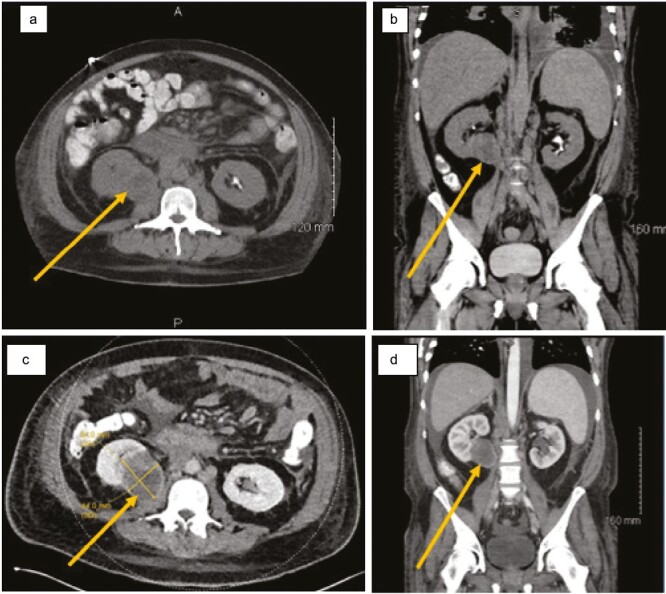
Computed tomography of right kidney mass at 2 timepoints. Axial (Panel A) and coronal (Panel B) CT at the time of initial workup demonstrated a mass in the right lower pole of the kidney, noted by the arrows, measuring 4.7 × 4.7 cm. Despite its considerable size, the mass was asymptomatic and, therefore, may have contributed to the challenge in diagnosing the cancer that was ultimately determined to be of renal origin by molecular testing. Axial (Panel C) and coronal (Panel D) CT approximately 3 weeks after his initial workup and diagnosis, with images taken just prior (coronal) and immediately following (axial) his transfer, demonstrated growth of the long axis of the right kidney mass to 6 cm, a rapid pattern of progression.
